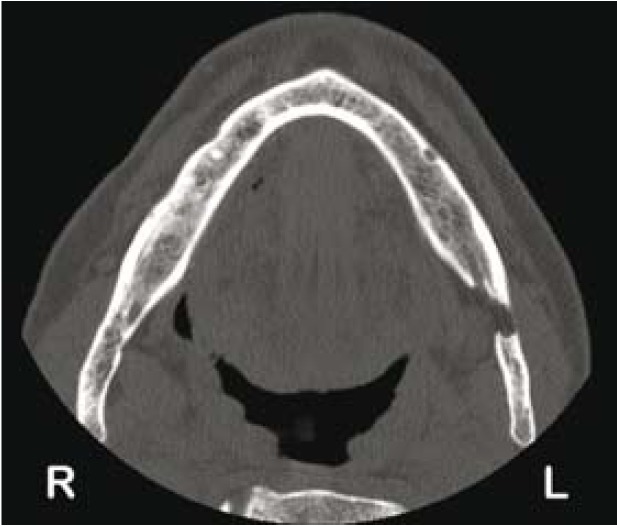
Axial section of CT scan at one year follow-up. No changes are observed with respect to the previous CT.
